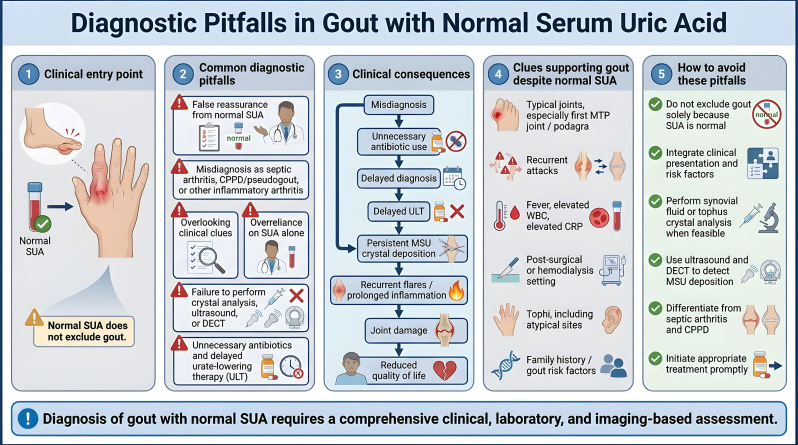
Diagnostic pitfalls in gout with normal SUA. This figure summarizes key challenges in diagnosing gout when SUA is normal, including misdiagnosis, unnecessary antibiotic use, and delayed ULT. Clinical clues such as typical joint involvement, recurrent attacks, fever, elevated WBC or CRP, post-surgical or hemodialysis setting, and tophi (including atypical sites) are highlighted. Strategies to avoid pitfalls include crystal confirmation, imaging (US, DECT), and timely initiation of ULT. SUA, serum uric acid; ULT, urate-lowering therapy; WBC, white blood cell count; CRP, C-reactive protein; MSU, monosodium urate; US, ultrasonography; DECT, dual-energy computed tomography.
